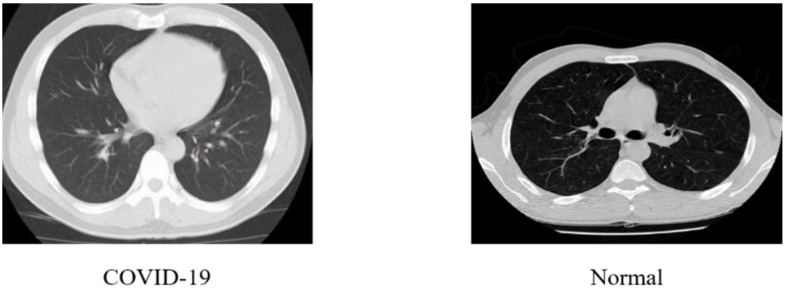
CT scan images of COVID-19 and healthy patients.
